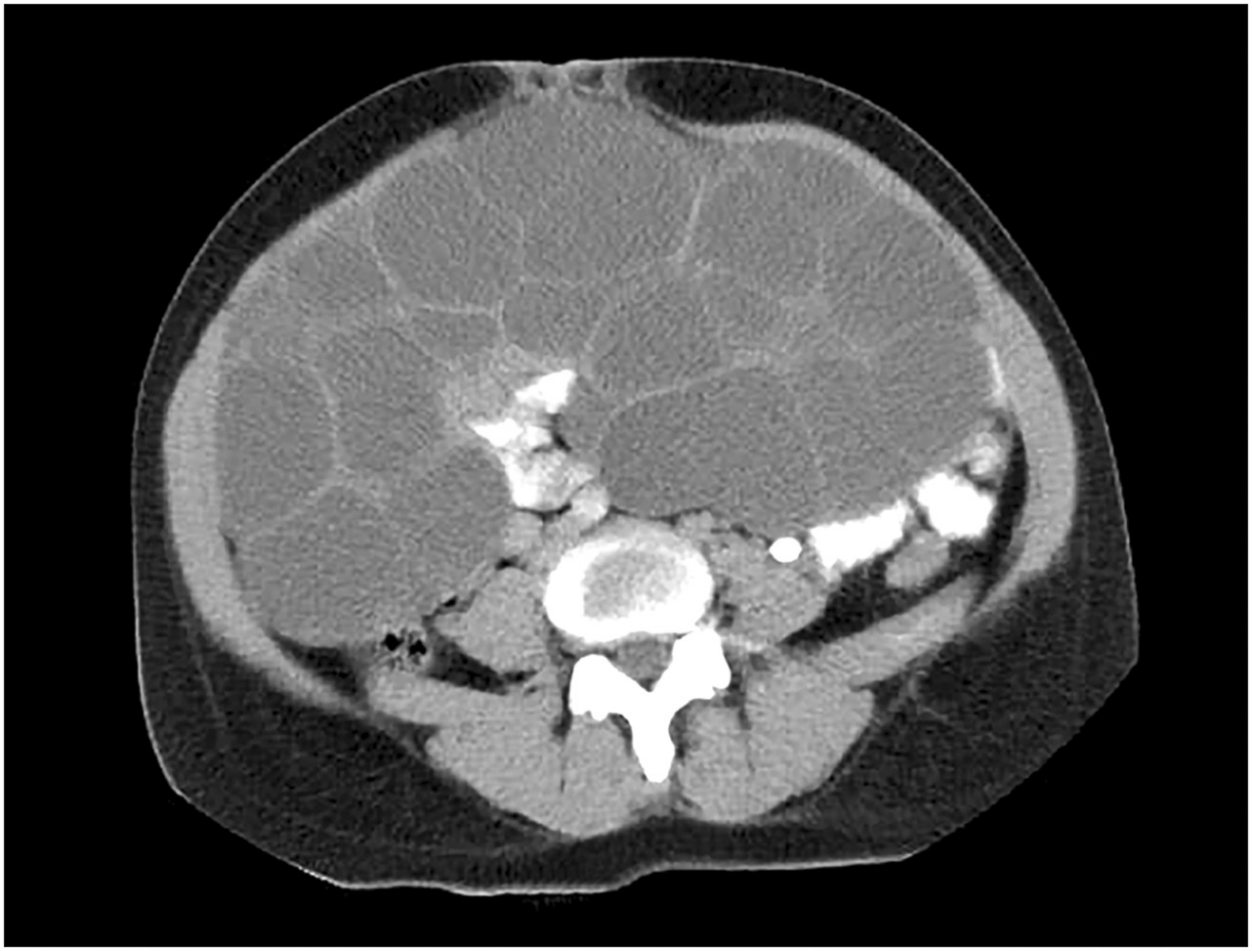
Axial view of the abdominal computed tomography in the venous phase with intravenous and oral contrast media showing a massive septated cystic complex of the ovaries that measures up to 40 × 15 × 23 cm. The structure fills the pelvis and extends to the epigastrium.
